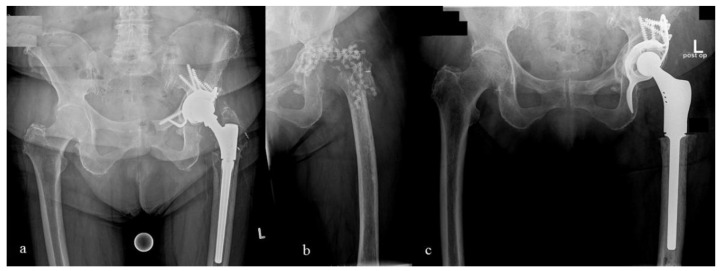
(a) Anterio-posterior radiographs of the pelvis of 78-year-old woman. Notice that anchors are already present in the major trochanter indicating a prior refixation; (b) During explantation, a refracture of the extremely weakened major trochanter occurred, so a resection arthroplasty was carried out instead of a spacer implantation; (c) Following an interim period of 54 days, the prosthesis reimplantation was performed (Burch-Schneider^®^ antiprotrusio cage, cemented polyethylene cup, Fa. ZimmerBiomet, Freiburg im Breisgau, Germany; GMRS^®^, Fa. Stryker, Duisburg, Germany).
